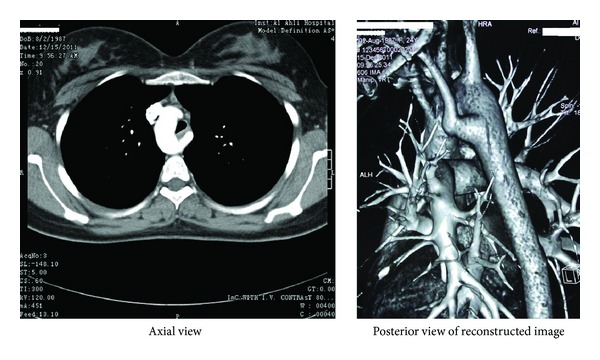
A collated picture of chest CT scan with an image of axial view and a reconstructed image with posterior facing showing a right-sided aortic arch, an aberrant origin of left Subclavian artery (Kommerell's diverticulum) behind the oesophagus which was obscured due to compression.
